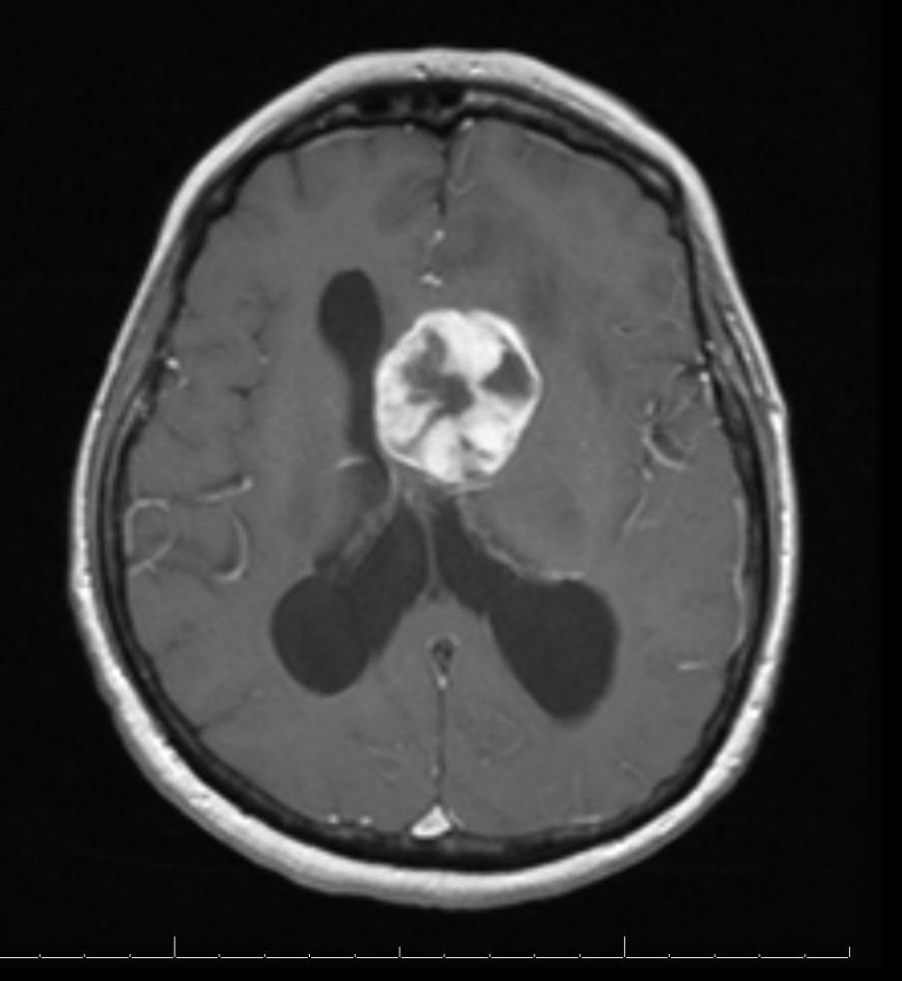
Initial MRI (post-contrast) T1 axial post-contrast MRI showing the heterogeneous enhancement of the lesion.
